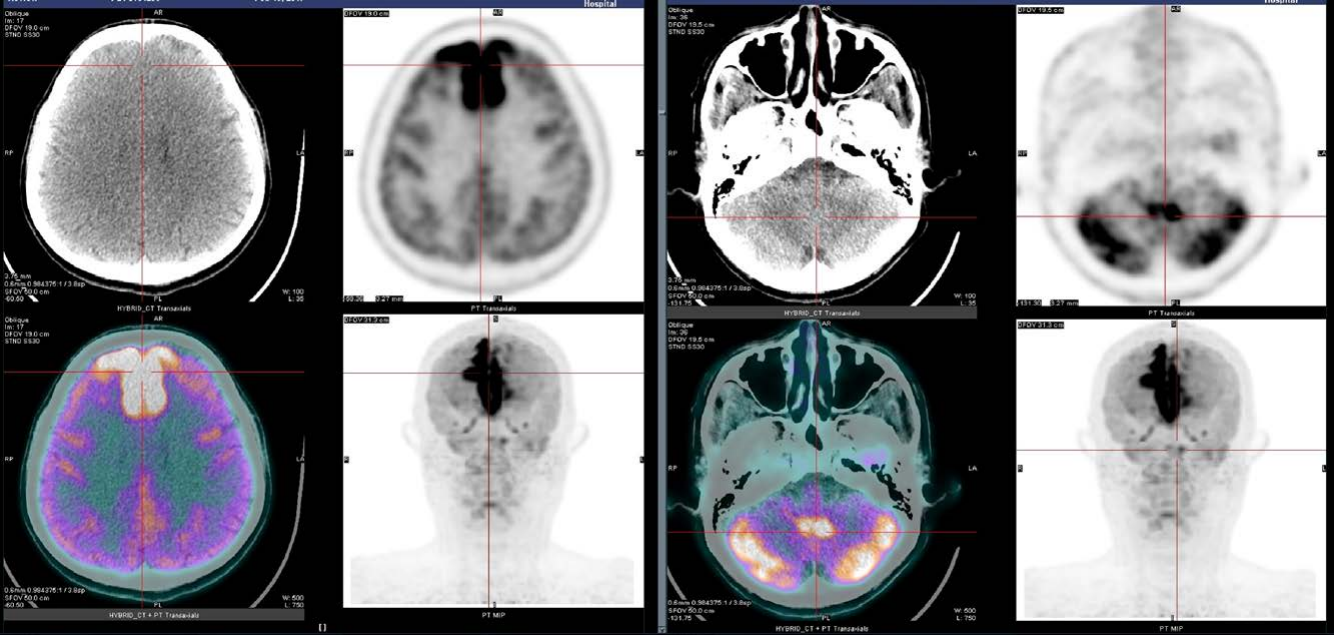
^18^F FDG PET/CT showed a symmetrical hypermetabolism in the bilateral medial frontal gyrus, cerebellar cortex, and dentate nucleus, especially in the bilateral frontal lobes. FDG = fluorodeoxyglucose, PET/CT = positron emission tomography/computed tomography.
